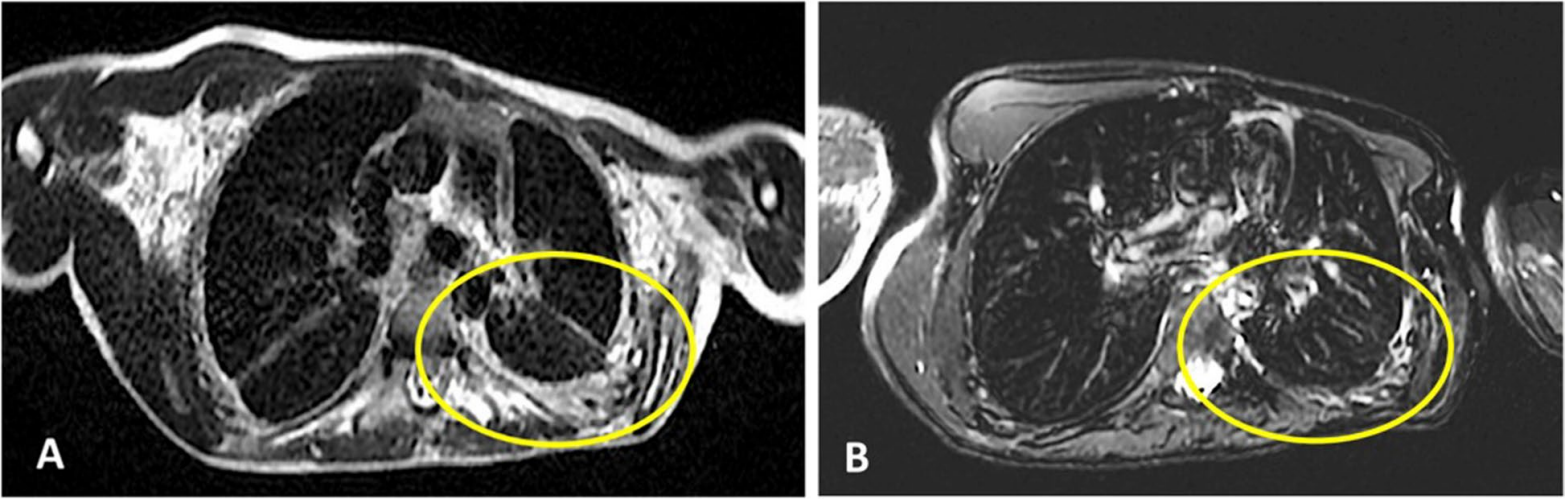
A Magnetic Resonance Imaging (MRI-T2) of thorax in 2018 (before starting sirolimus): pleural thickening (yellow circle). B MRI of thorax in 2022 (on sirolimus + trametinib): reduction of pleural thickening
